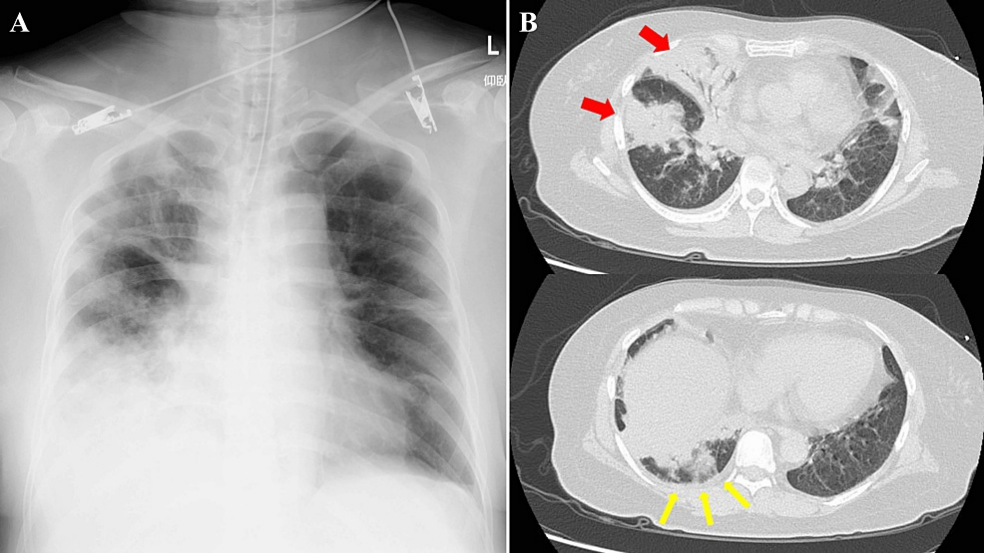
Chest radiography and CT images for case 2. (A) Chest radiography upon admission for the patient in case 2 revealing consolidation in the right upper and lower lung fields. (B) Chest CT image upon admission for the patient in case 2 revealing extensive consolidation throughout the right lobe (red arrows) and very few pleural effusions in the right thoracic cavity (yellow arrows).
